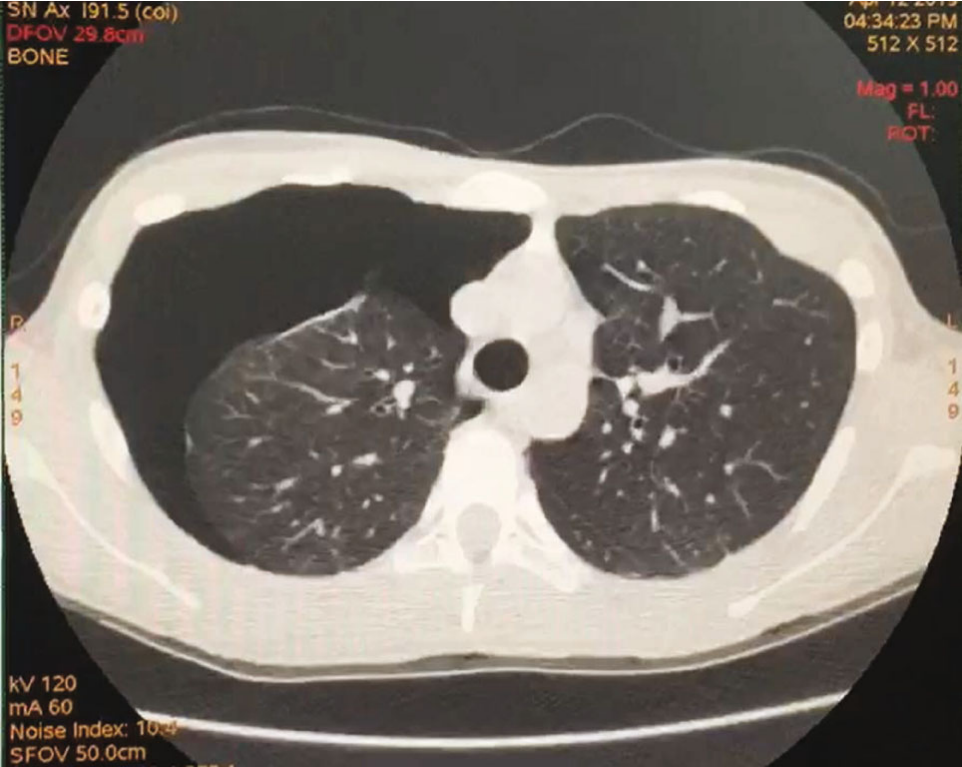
Noncontrast chest CT scan demonstrates the presence of pneumothorax on the right hemithorax.
